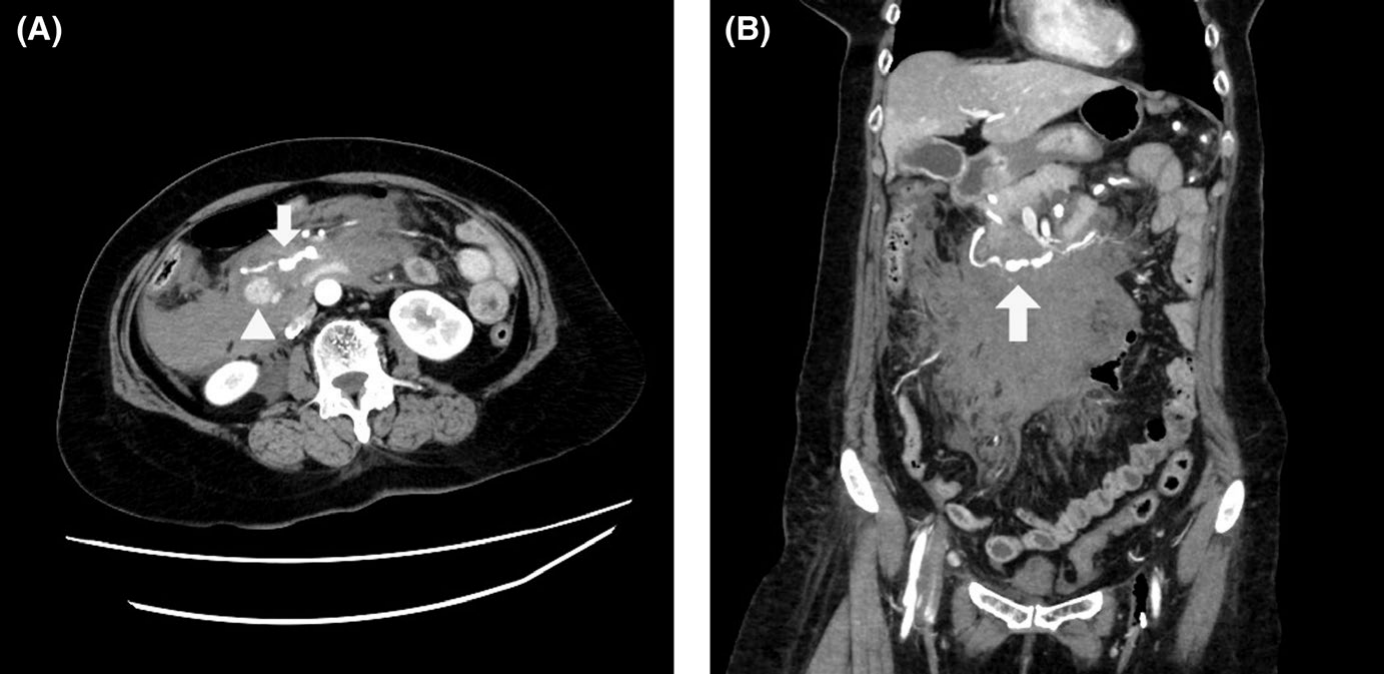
A, Enhanced CT scan showed ruptured PDA aneurysm (arrow) and extravasation (arrowhead). B, Enhanced CT scan (coronal view) showed ruptured PDA aneurysm (arrow) and massive retroperitoneal hematoma
